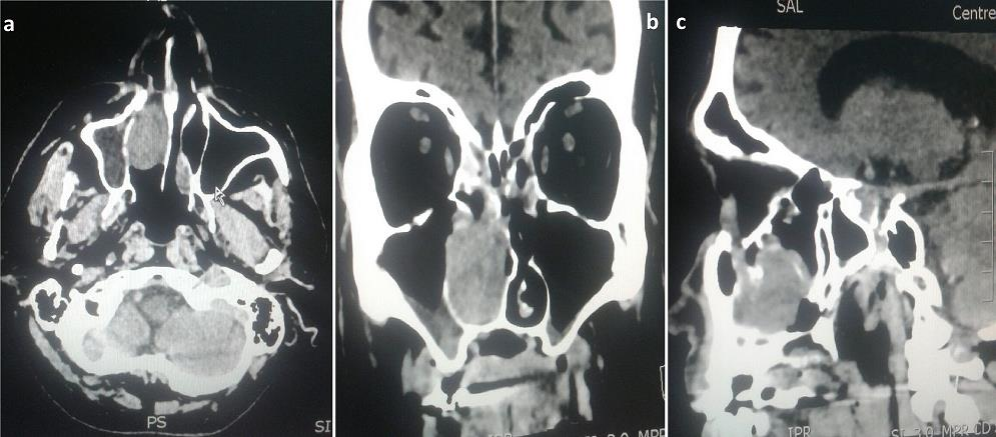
Sinonasal computed tomography (CT) viewed at soft tissue windows shows a right nasal mass filling the right-side nasal cavity enhanced after injection of contrast product: (A) axial post-contrast CT; (B) coronal post-contrast; (C) sagittal post-contrast CT
